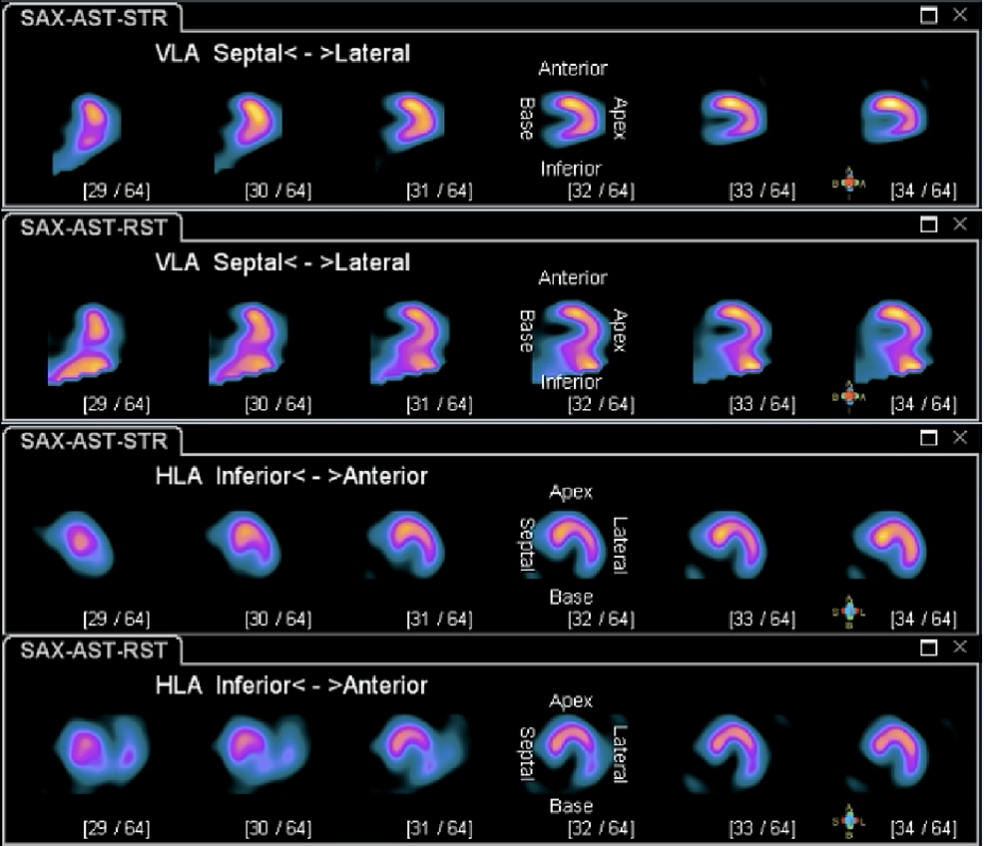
SPECT image 3 showing a small to moderate reversible perfusion defect present in the mid and basal aspect of the inferoseptal wall of the left ventricle. SPECT: single photon emission computed tomography.
